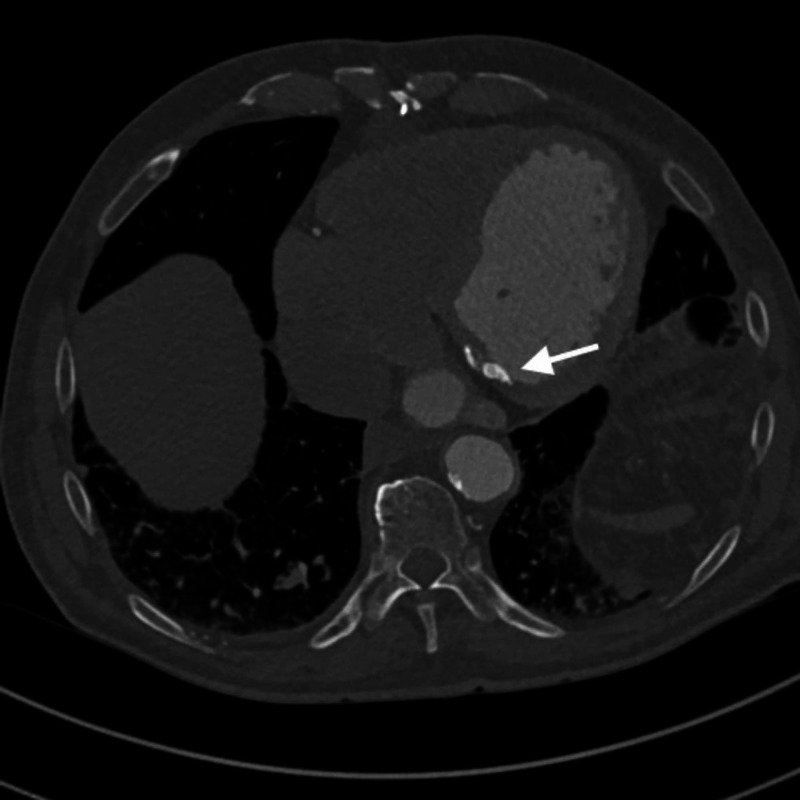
Axial contrast-enhanced coronary CT at the level of the mitral annulus demonstrates hyperdense calcific deposits consistent with annular calcifications.
